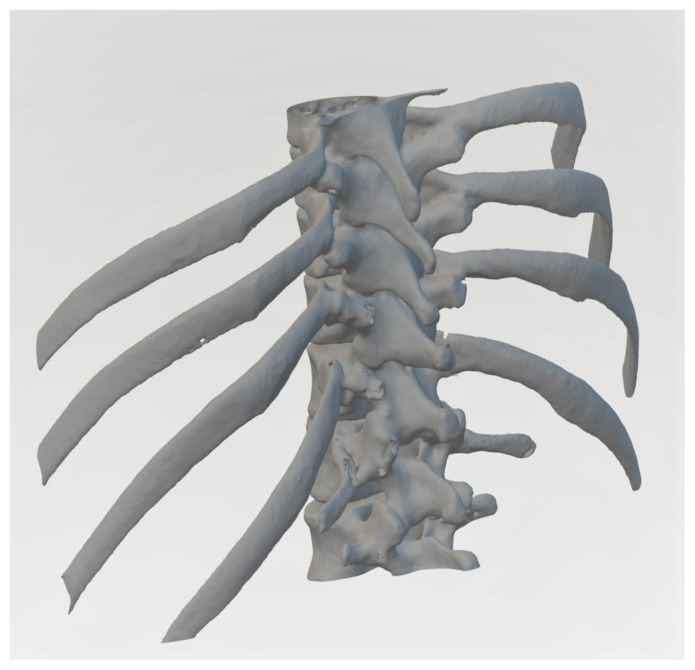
Prevue Medical. Lower Spine and Ribcage from Chest CT by Prevue. In: Thingsverse. https://www.thingiverse.com/thing:54669/files. Published April 7, 2013. Accessed April 15, 2024. CT scan file of the segment of spine and ribcage used to create the model. The file was further modified to reduce the size, which involved cropping of the two inferior vertebrae and shortening of the ribs by approximately half the length.Upon completion of the 3D printing process (approximately 22 hours), the model was then placed into a hot water bath to remove the external support material. The model was then additionally washed with soap and water to remove any remaining debris.[Fig f2-jetem-10-2-i1]Figure 2 f2-jetem-10-2-i1:
